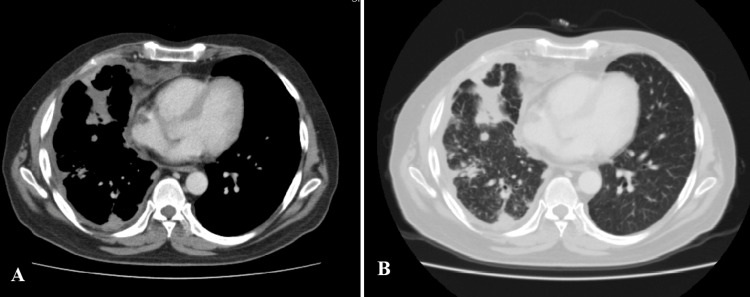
(A) CT chest with contrast showing the collection in and beyond the thoracic cage. (B) CT chest with contrast (lung parenchymal window) showing consolidation in the right upper lobe and nodular infiltrates.
